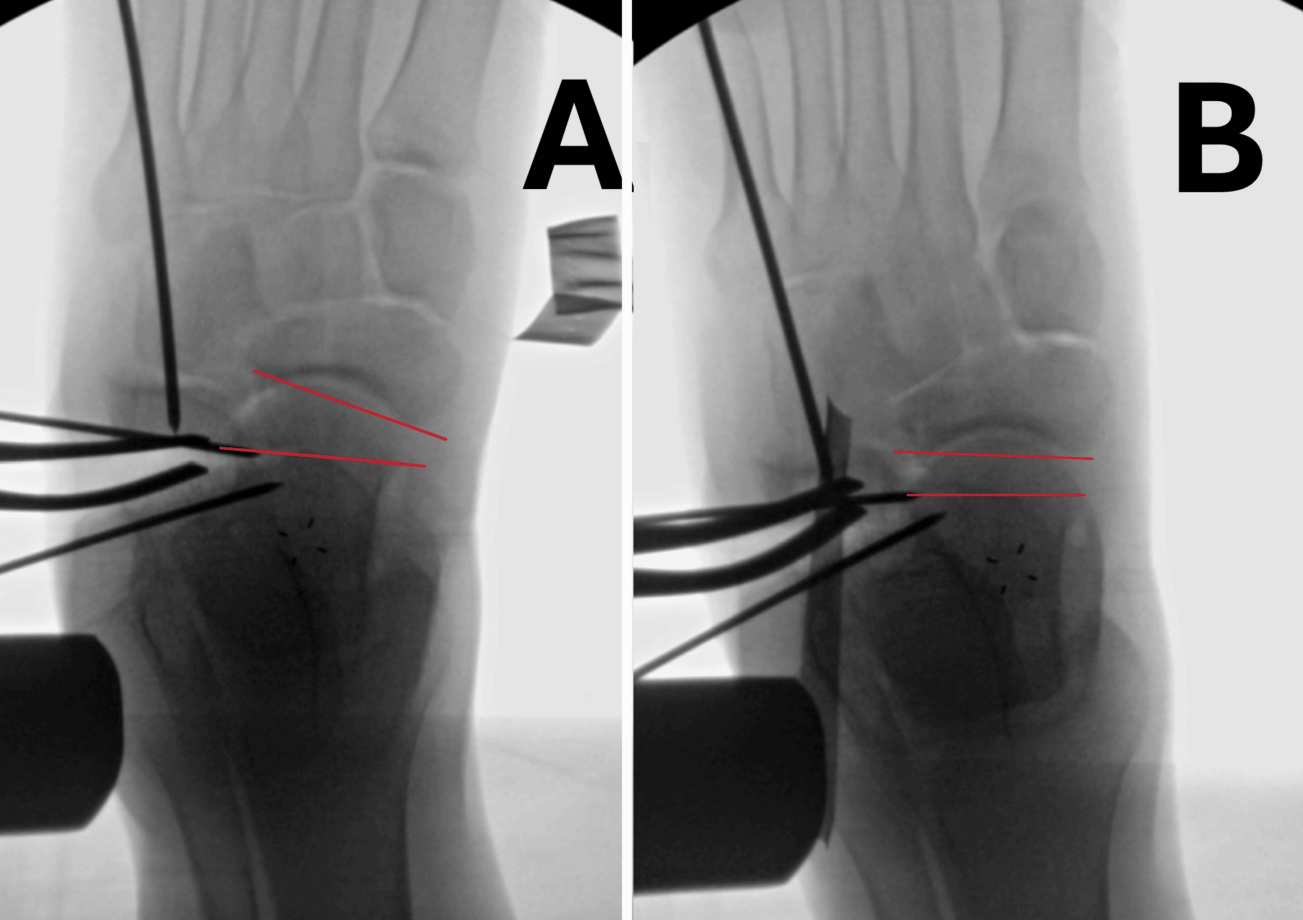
Intraoperative x-ray images showing correction of talonavicular coverage angle. (A) During osteotomy distraction before applying dorsiflexion and eversion stress. (B) The joint is under stress, with red lines indicating the articular surfaces of the talar head and navicular.
